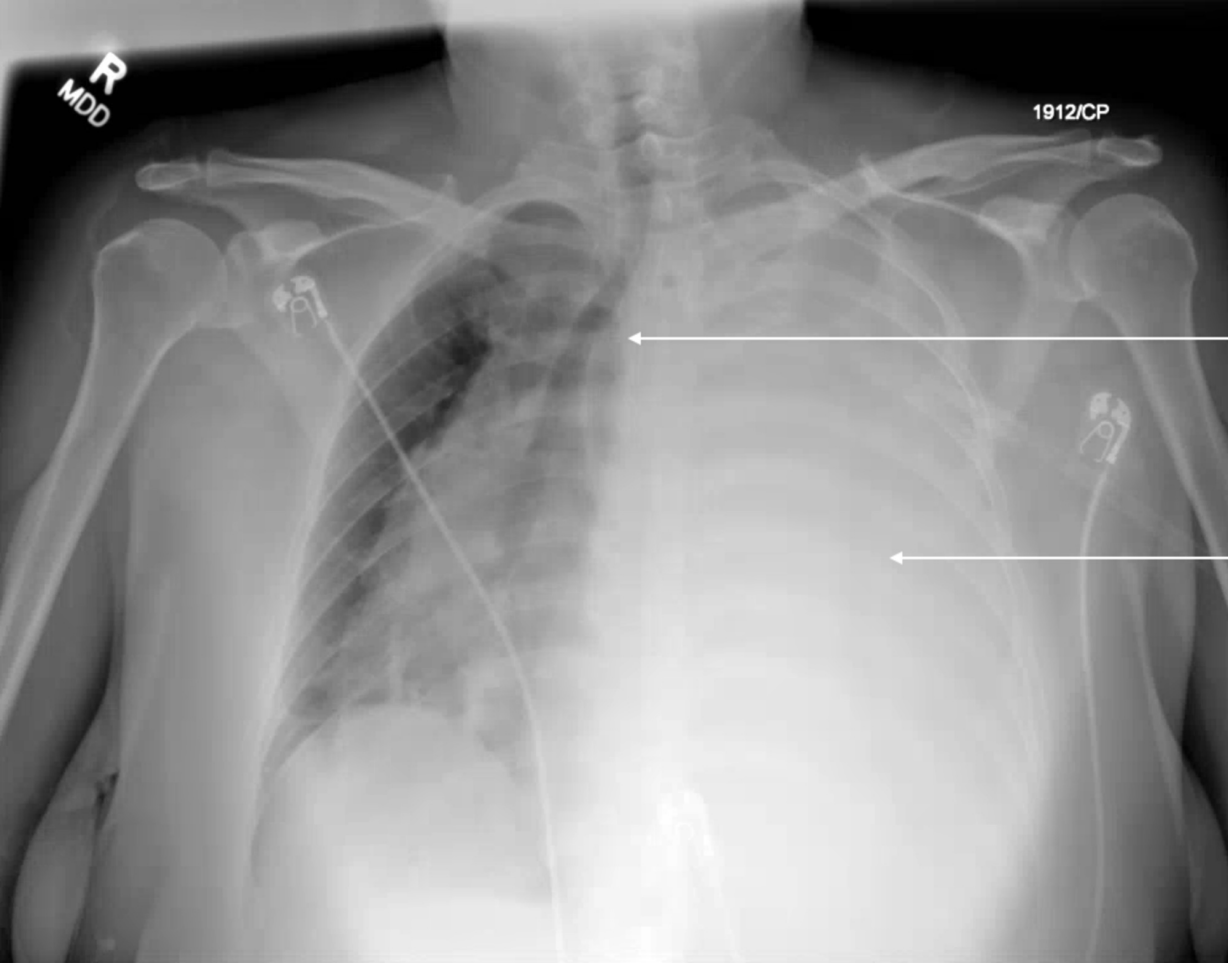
Chest x-ray showing complete left hemithoracic opacification with mass effect including rightward mediastinal shift.
